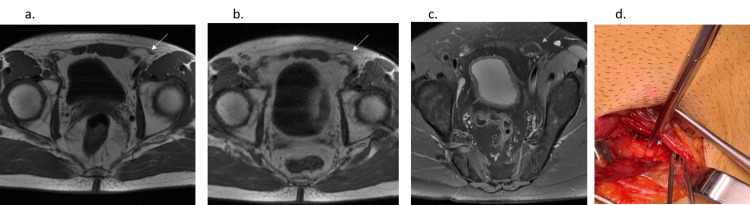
A 28-year-old man, professional tennis player with left pain. Axial T1 in AW-related Chronic Groin Pain (CGP) (a) and Axial oblique Proton Density weighted with Fat Saturation (PDFS) in Abdominal Wall (AW) (c) with bulging (fatty overload): grade 3 in left inguinal orifice (white arrow) in these static MRI sequences. This AW lesion becomes grade 4 in the left inguinal orifice in axial T1 VM (b) (same patient, same level). Left AW exposed in AW-related CGP (before the Shouldice technique) with black arrow = weakness at conjoint tendon insertion on left Raectus Abdominis.
